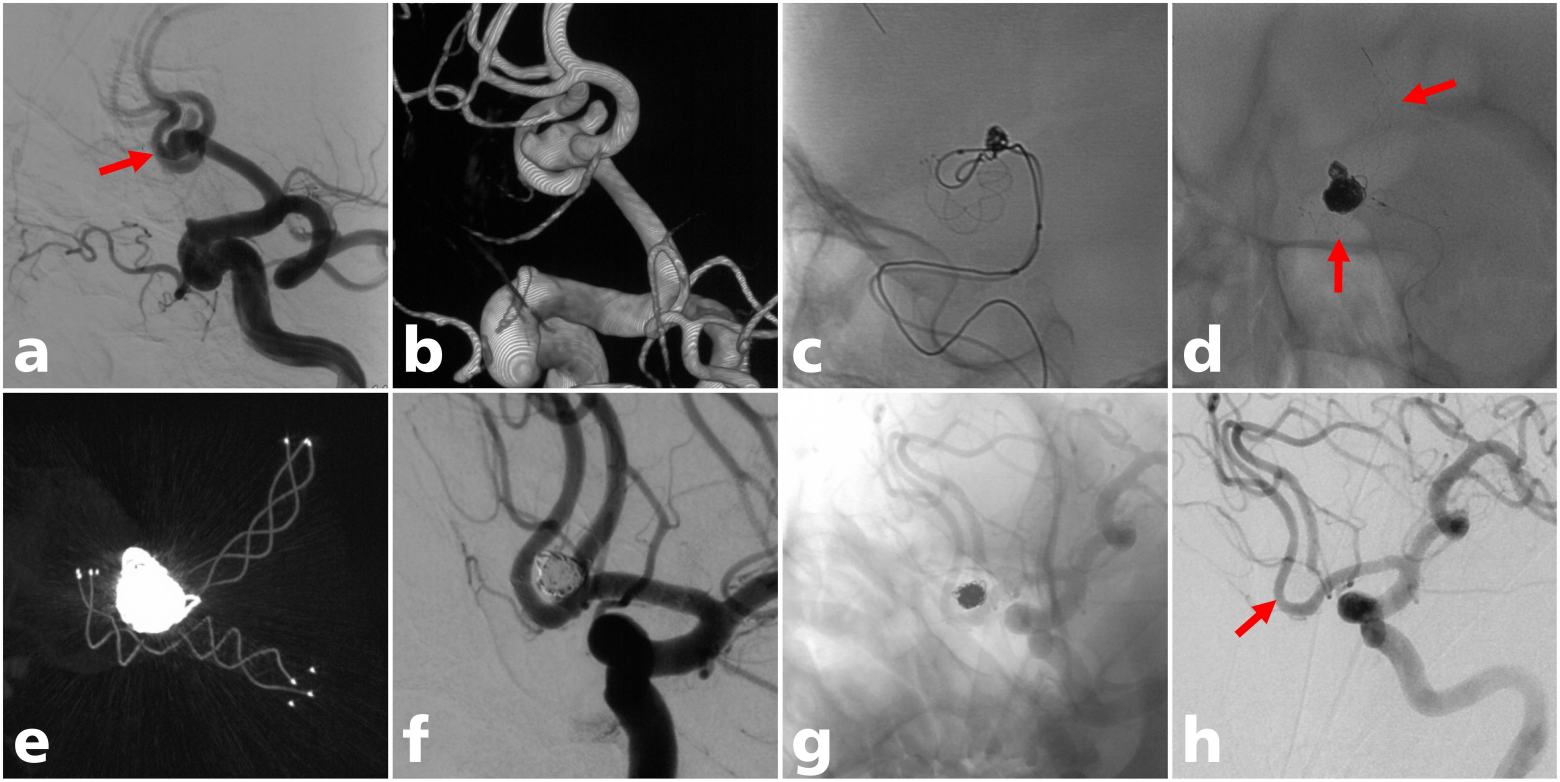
A 60-year-old man with an ACoA aneurysm treated with LVIS stent-assisted coiling with a T configuration. (a) Angiogram showed a wide-neck ACoA aneurysm (solid arrow); (b) three-dimensional reconstruction of the aneurysm; (c) the LVIS stent (3.5 mm × 20 mm) was successfully deployed crossing the ACoA to the contralateral A2 segment; (d) the LVIS Jr stent (3.5 mm × 18 mm) was successfully deployed from the ipsilateral A2 to the A1 segment in a T configuration (solid arrow); (e) postoperative CT reconstruction showed that the two stents were complete opened (f) partial occlusion (modified Raymond-Roy class IIIa) was achieved under final view; (g,h) complete occlusion (modified Raymond-Roy class I) of the aneurysm at last angiographic follow-up (solid arrow).
